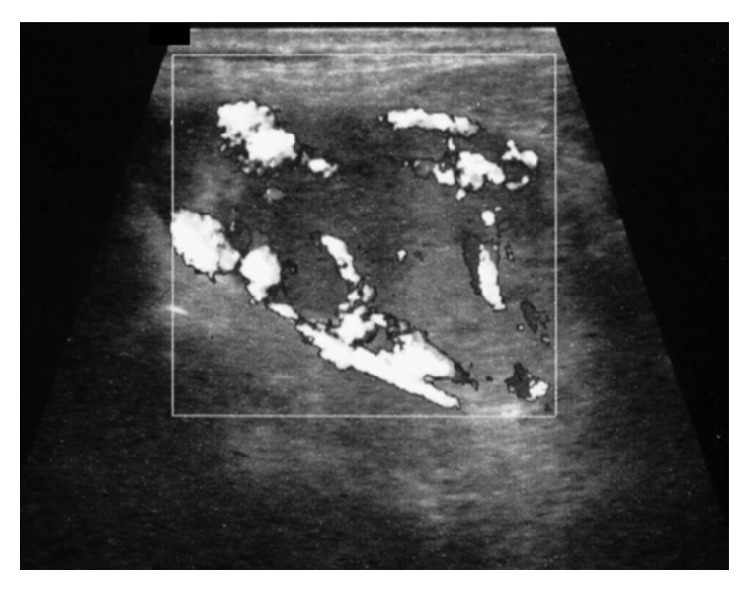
Thyroid ultrasonography showing a left 5 cm solid and isohypoechoic nodule with peripheral and intranodular vascularization.
